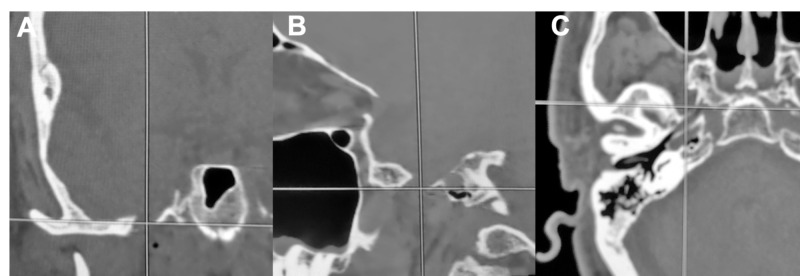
Coronal (A), Sagittal (B), and Axial (C) CT images with superimposed cross-hair marking the intended target for needle entry into the foramen ovale.
